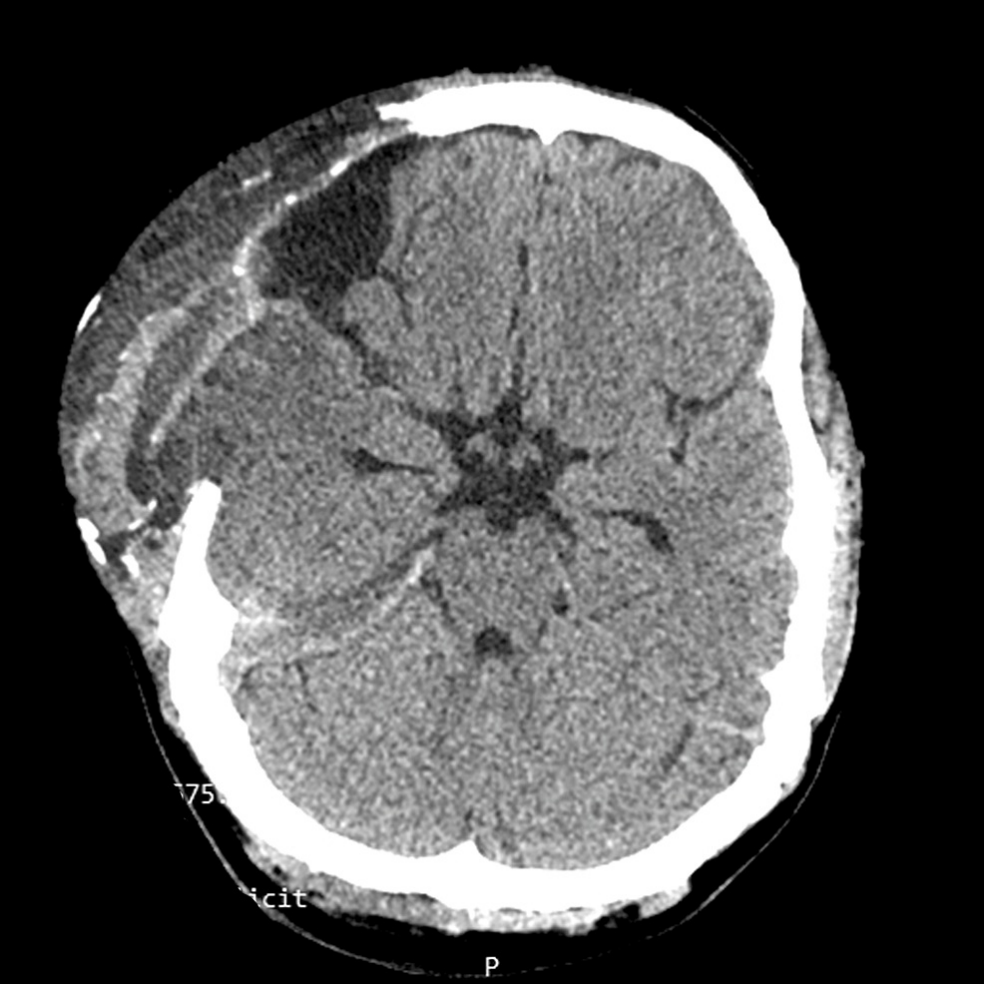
Post-operative CT showing residual clots with significant reduction in cerebral edema.
